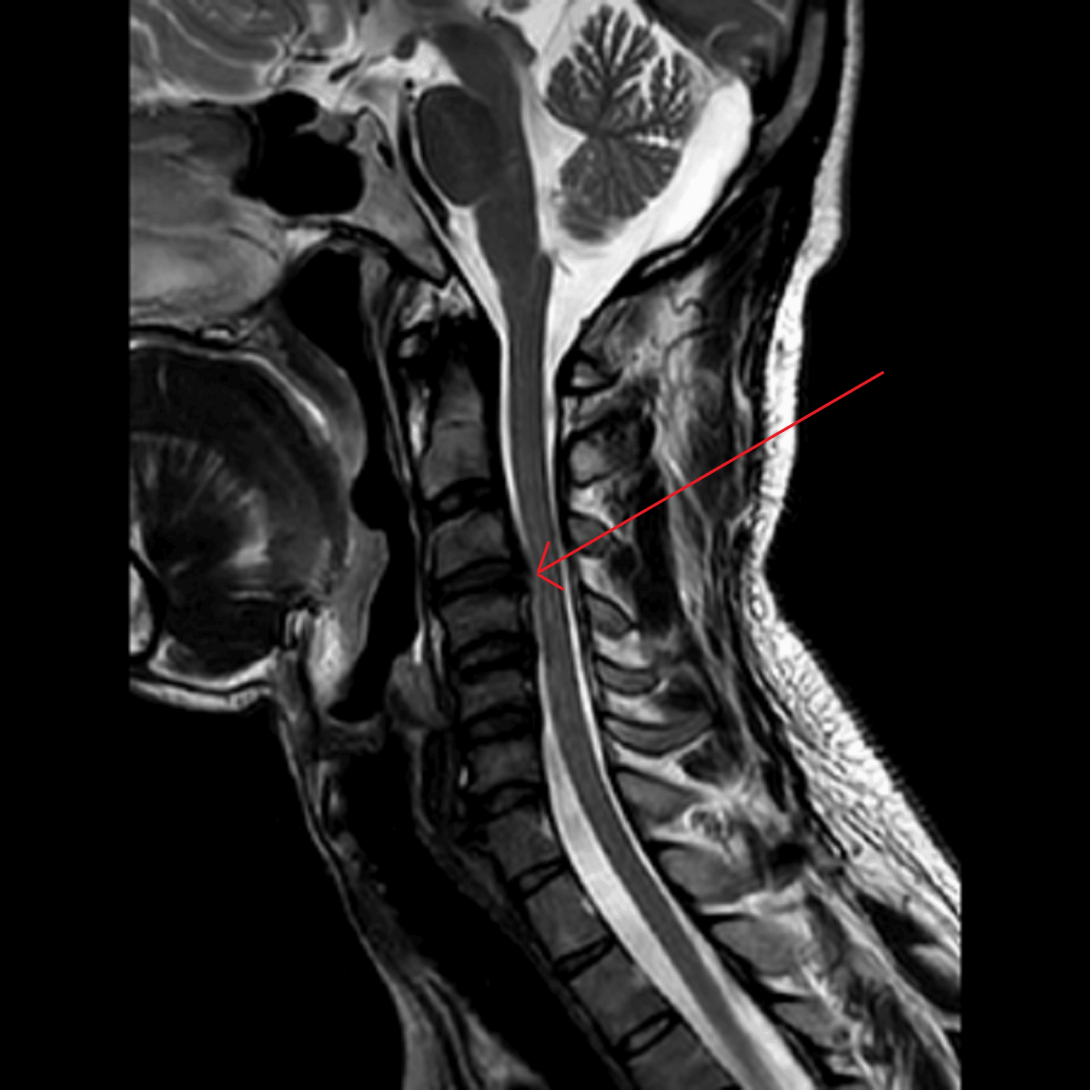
MRI brain and spine The arrow indicates a focal left para-central disc protrusion- small hernia, contacting with, and slightly compressing the spinal cord. The was no evidence of demyelination or cord signal change MRI: magnetic resonance imaging
